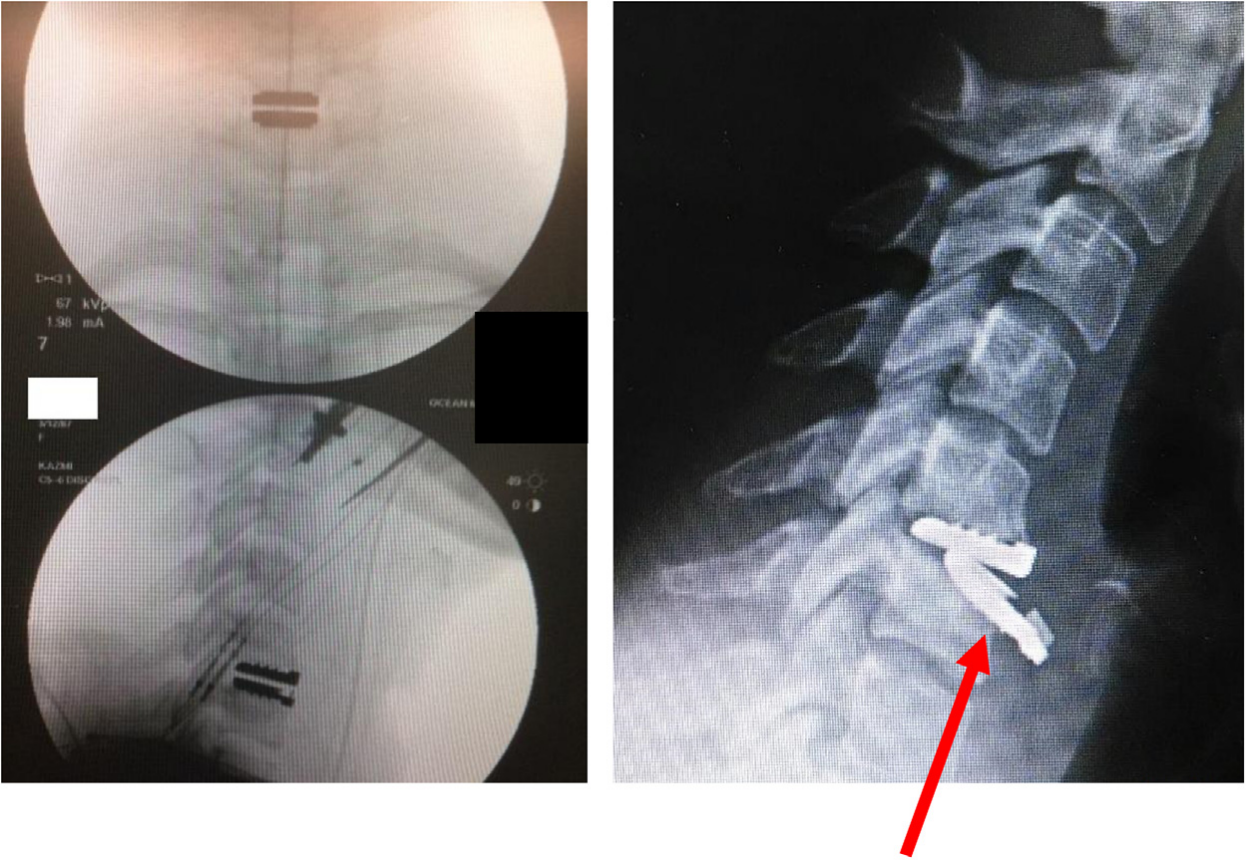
(Left) Intraoperative and (Right) preoperative radiographs with the arrow indicating anterior migration of the inferior component with the convex UHMWPE surface.
